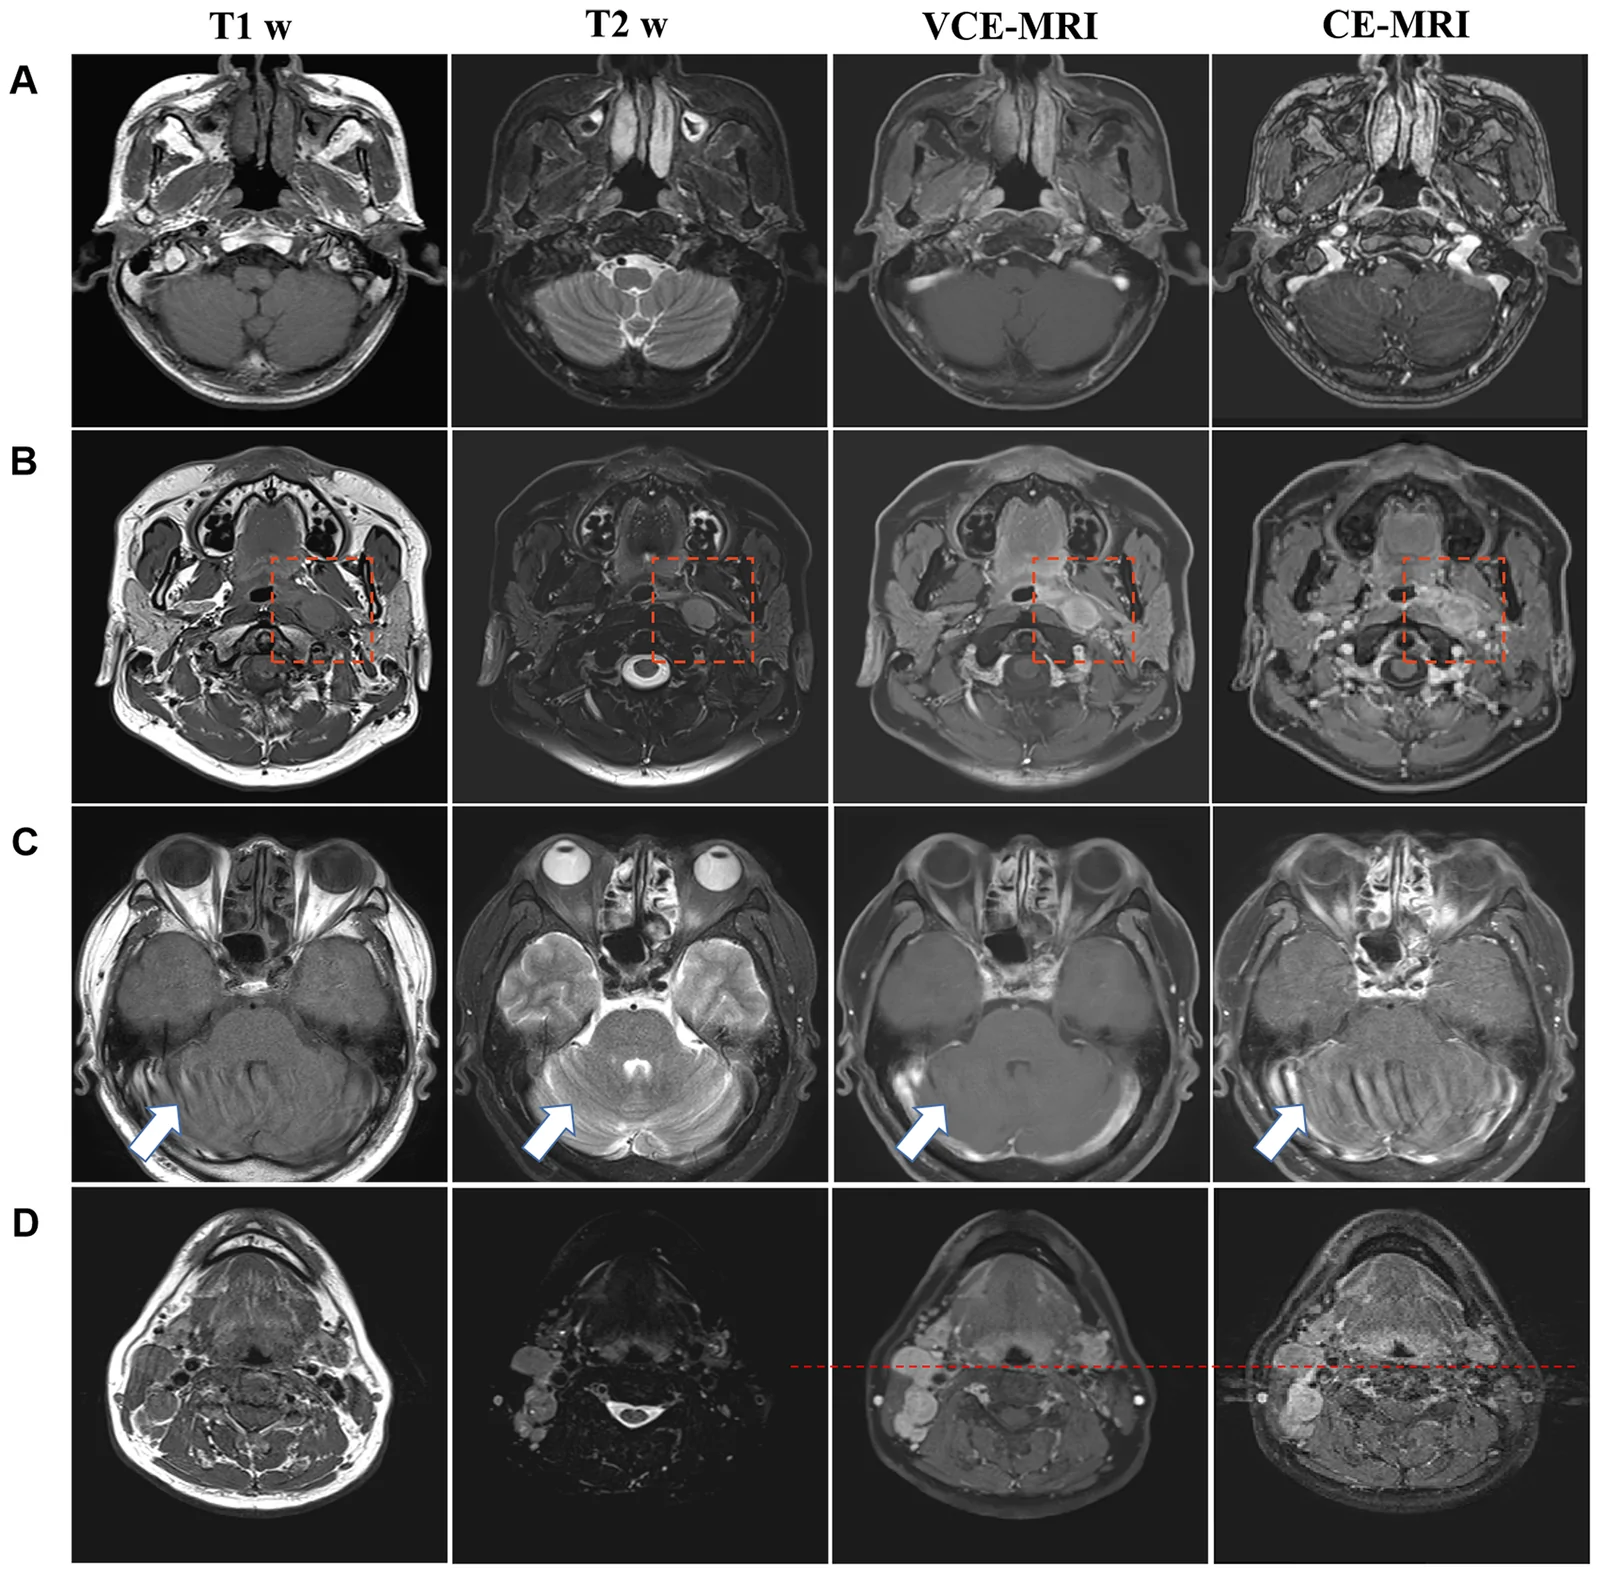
Example NPC MRI from the study. From left to right: T1-weighted images, T2-weighted images, virtual contrast-enhanced MRI(VCE-MRI) and contrast-enhanced MRI(CE-MRI). (A) The noise in the VCE-MRI images was significantly reduced compared to the CE-MRI. (B) The noise reduction in the VCE-MRI images was accompanied by clearer boundaries of the tumor and surrounding structures. (C) The VCE-MRI notably reduced the artifacts in the brain area. (D) There was a marked displacement in the pharynx and lymph nodes on the VCE-MRI compared with input images and CE-MRI.
